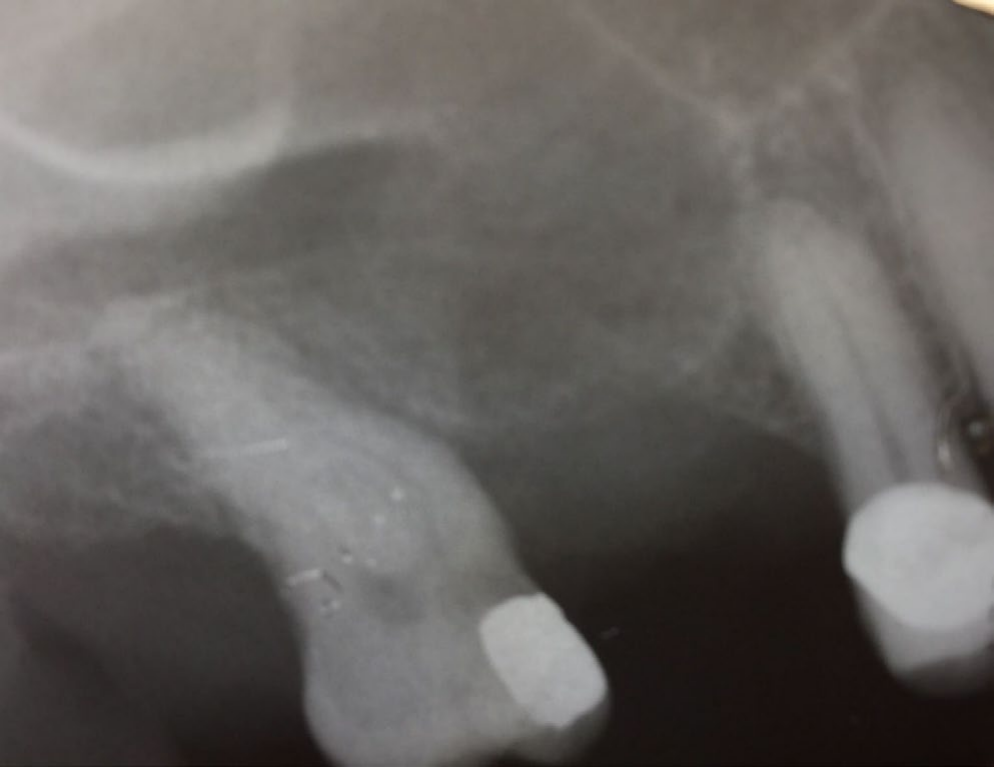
Radiograph of the treated site
